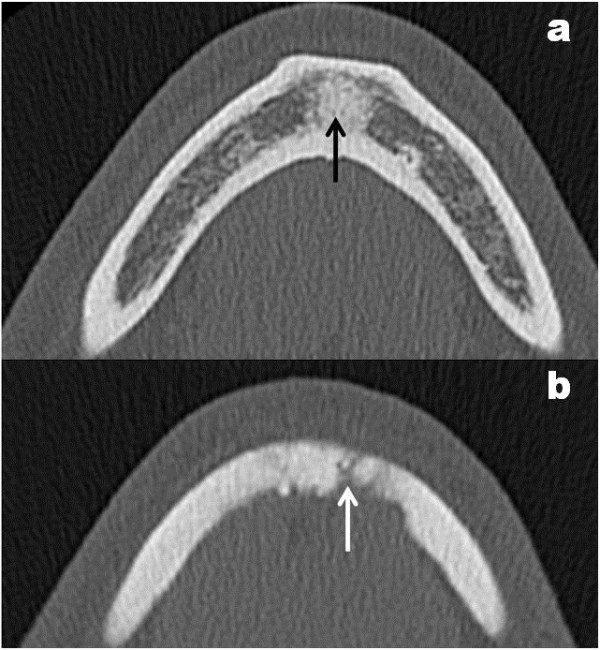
Computed tomography axial scans of the mandible. Computed tomography axial scans of the mandible show (a) the aspect of the thickening osteitis of the anterior arch (black arrow) and (b) the irregular bone resorption (white arrow).
